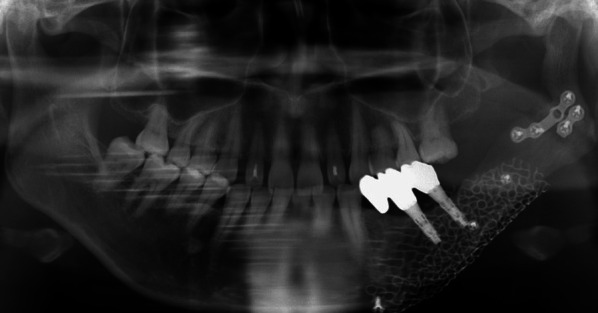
Panoramic radiograph after prosthetic treatment
